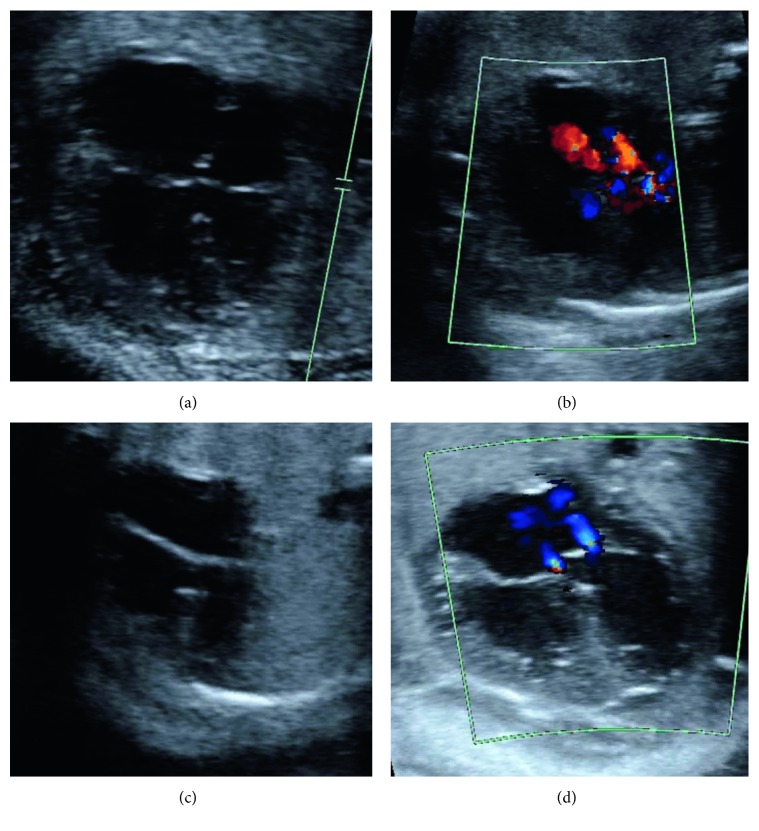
Fetal four-chamber cardiac images. (a) Twin A 2D imaging showing a ventricular septal defect, primum atrial septal defect, and secundum atrial septal defect. (b) Twin A color Doppler showing left and central atrioventricular valve regurgitation (red flashes moving toward the transducer). (c) Twin B 2D imaging showing a ventricular septal defect, primum atrial septal defect, and secundum atrial septal defect (drop out artifact between these defects gives the incorrect appearance of a large single defect). (d) Twin B color Doppler showing left and central atrioventricular valve regurgitation (blue flashes moving away from the transducer).
